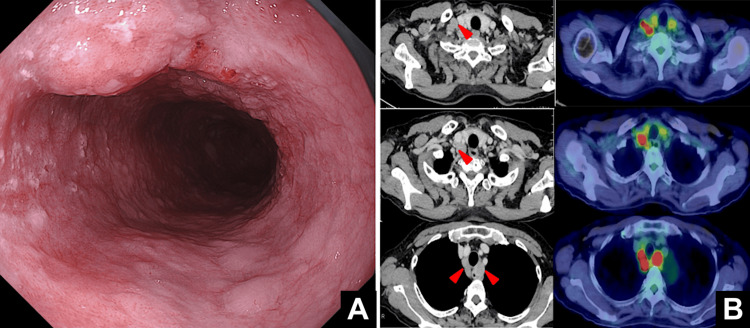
Pre-treatment imaging findings. (A) Pre-treatment endoscopic image showing an elevated lesion in the upper thoracic esophagus. On the anal side of this lesion, a type 0-IIa + IIc lesion extended over approximately four-fifths of the luminal circumference. (B) Pre-treatment CT (left) and PET-CT (right) images showing the right supraclavicular lymph node, the 106recR lymph node, and the 106recR/106recL lymph node enlargement with FDG uptake, indicating lymph node metastasis. The enlarged lymph nodes are indicated by red arrowheads on the images.
